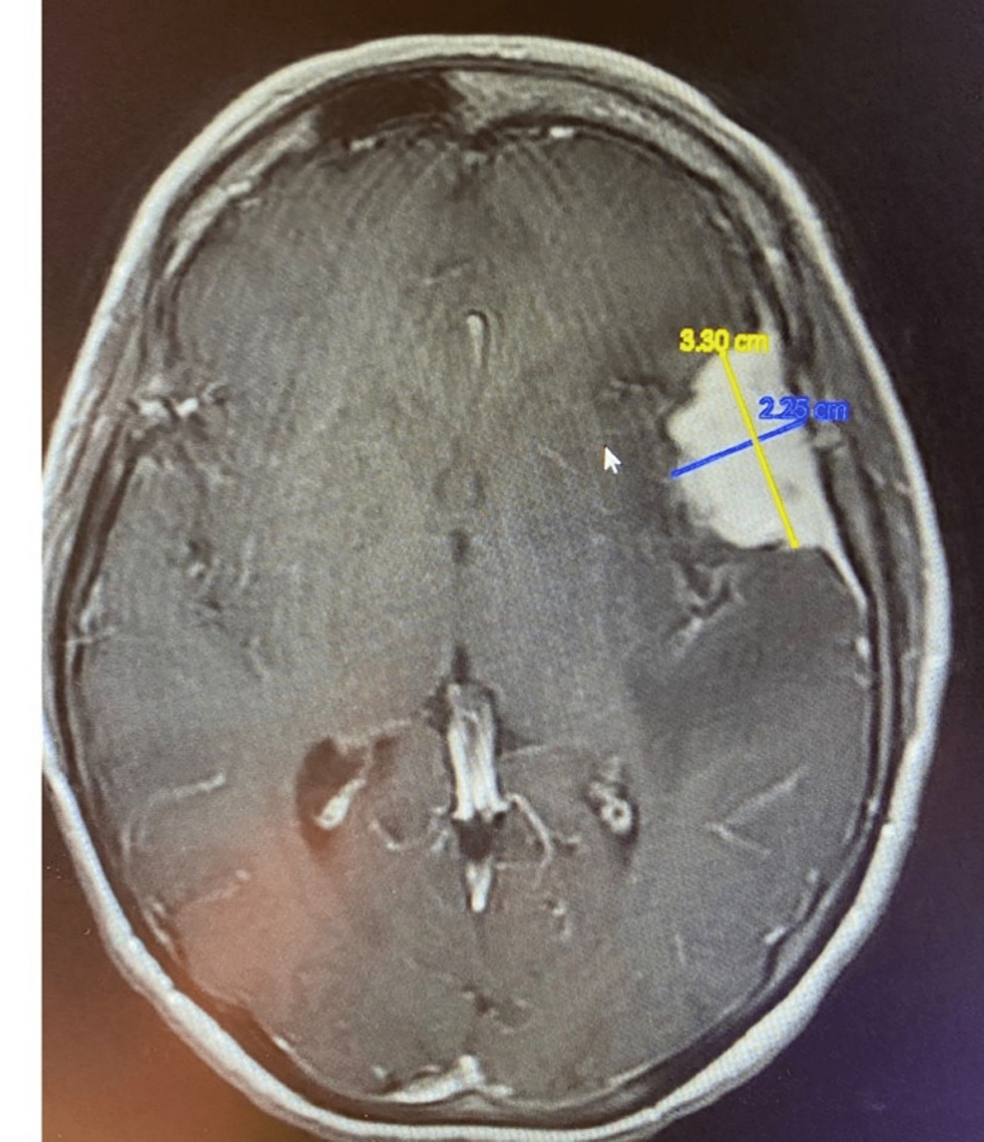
MRI showing left anterior middle fossa meningioma
